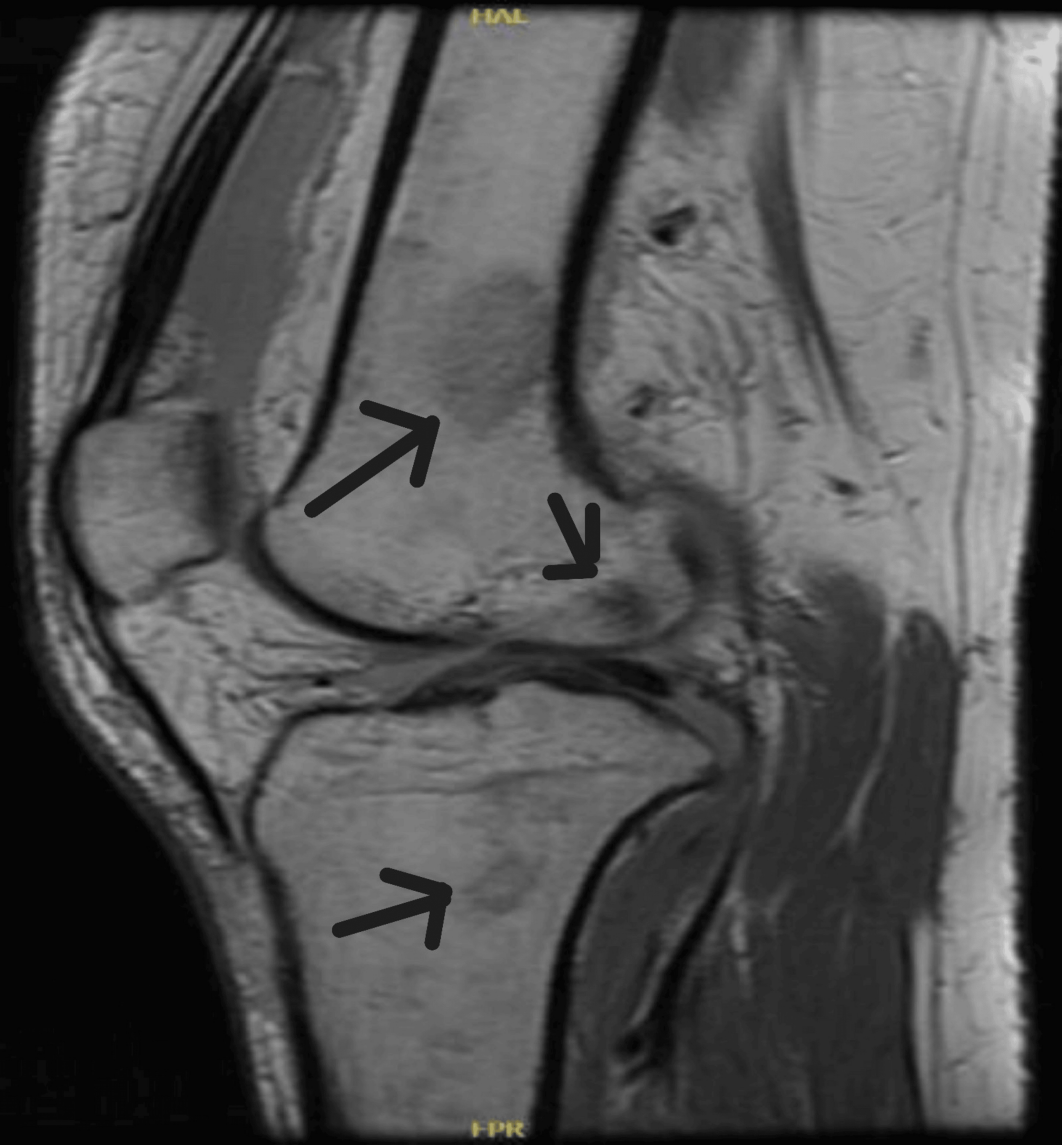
MRI knee: marrow signal abnormalities involving the distal femur and proximal tibia MRI, magnetic resonance imaging
